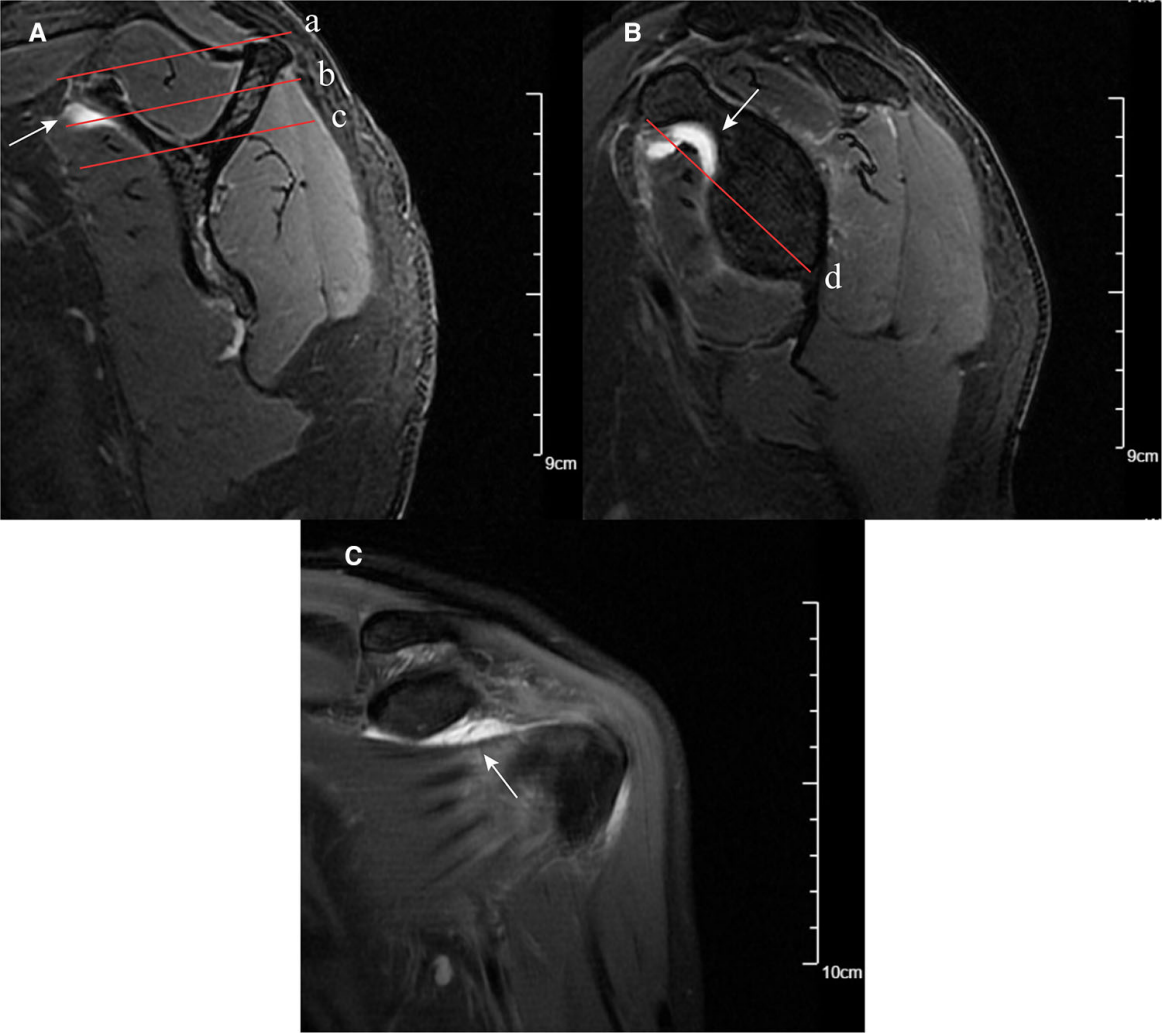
MRI images of subscapular muscle atrophy, effusion, and fluid area ratio (en-face). (A) SSC muscle atrophy classification on the Y-face plane. Parallel line intercepting supraspinatus fossa opening (red solid line a) and parallel line (red solid line b) bisecting the perpendicular distance between line (red solid line a) and line (red solid line c). Based on these lines, the SSC position was classified: between lines (a) and (b) was classified as grade (I), between lines (b) and (c) was classified as grade (II), and below line (c) was classified as grade (III). Effusion on Y-face plane (white arrow). (B) En-face plane showing the base to-tip line (BTL): the inferior pole of glenoid to coracoid tip on en-face (red solid line d). Using BTL, muscle atrophy of SSC is classified as grade I (tendon and muscle exist above the BTL), grade II (only tendon exists above the BTL), and grade III (tendon and muscle exist below the BTL). The fluid area ratio was the ratio of the effusion area to the area surrounded by the coracoid process, glenoid, and BTL in the en-face. Effusion on en-face plane (white arrow). (C) Effusion on the coronal plane (white arrow).
